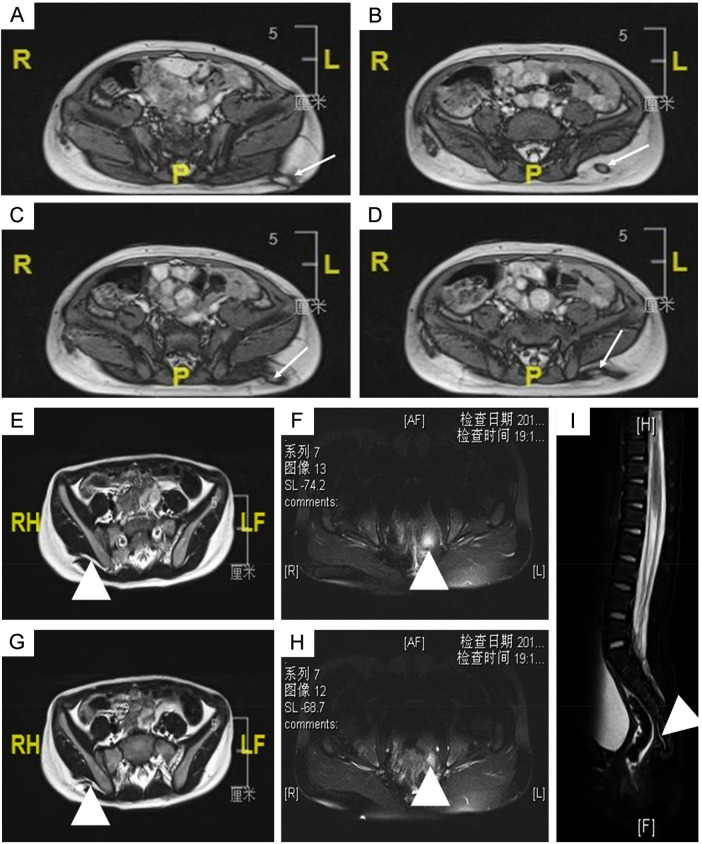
MRI imaging of congenital gluteal dermal sinus tract: (A–H), axial MRI images of children with congenital gluteal dermal sinus tract, with arrows and triangles indicating the location of the fistula in different sequences; (I), sagittal MRI image of children with congenital gluteal dermal sinus tract.
